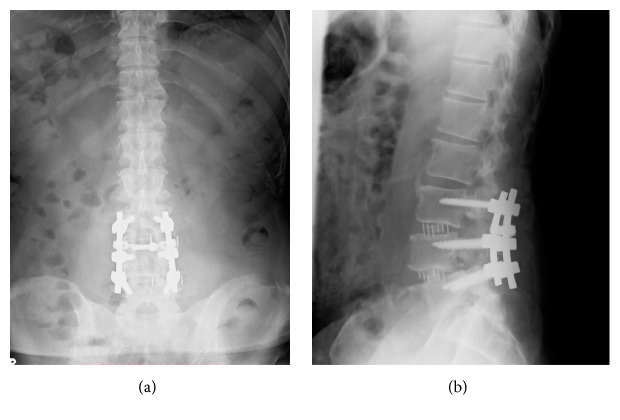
Postoperative radiography of the lumbar spine. AP view (a); lateral view (b). Lateral lumbar interbody fusion of L3/4 and L4/5 was performed at the initial surgery, and posterior decompression fixation using pedicle screws from L3 to L5 was performed at the second surgery.
